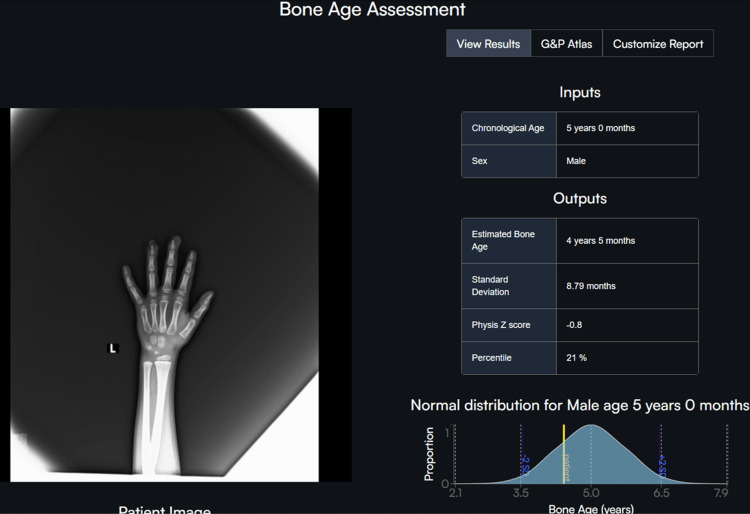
Automated bone age assessment output generated by the 16-Bit Physis platform. The figure demonstrates the 16-Bit Physis software interface displaying automated bone age estimation from the same left hand radiograph of a five-year-old child. The platform provides skeletal maturity assessment and the corresponding bone age estimate derived using its artificial intelligence-based algorithm.
